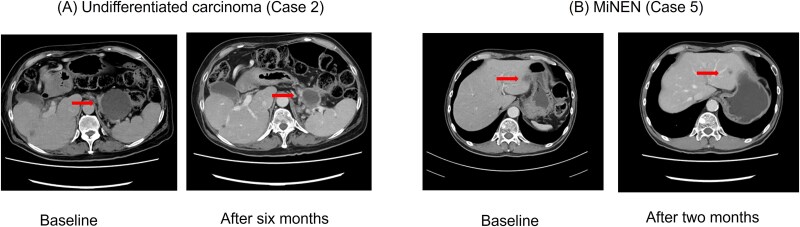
Representative CT images of cases that showed PR. (A) Undifferentiated carcinoma (case 2): Baseline CT obtained before the initiation of treatment with nal-IRI plus 5-FU and LV showed a pancreatic mass (→). Follow-up CT obtained after six months of treatment demonstrated marked tumor shrinkage consistent with partial response. Serum CA19–9 and CEA levels at the baseline were 381 U/mL and 9.3 ng/mL, respectively, the values decreased to 78.7 U/mL and 3.9 ng/mL, respectively, at six months after the start of treatment, when PR was achieved. (B) MiNEN (case 5): Baseline CT obtained before the initiation of treatment with nal-IRI plus 5-FU and LV showed one of multiple hepatic metastatic lesions (→). Follow-up CT obtained after two months of treatment demonstrated marked regression of this representative lesion, consistent with partial response. Serum CA19–9 and CEA levels at the baseline were 329 U/mL and 24.1 ng/mL, respectively; the values changed to 8.2 U/mL and 30.7 ng/mL, respectively, at two months after the start of treatment, when PR was confirmed.
